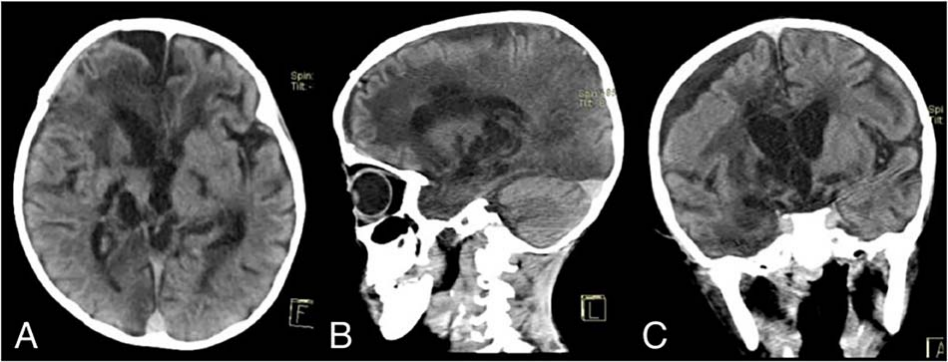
Discharge noncontrast head CT scan showing that the abscess has been completely removed; (A) axial view; (B) sagittal view; (C) coronal view.
